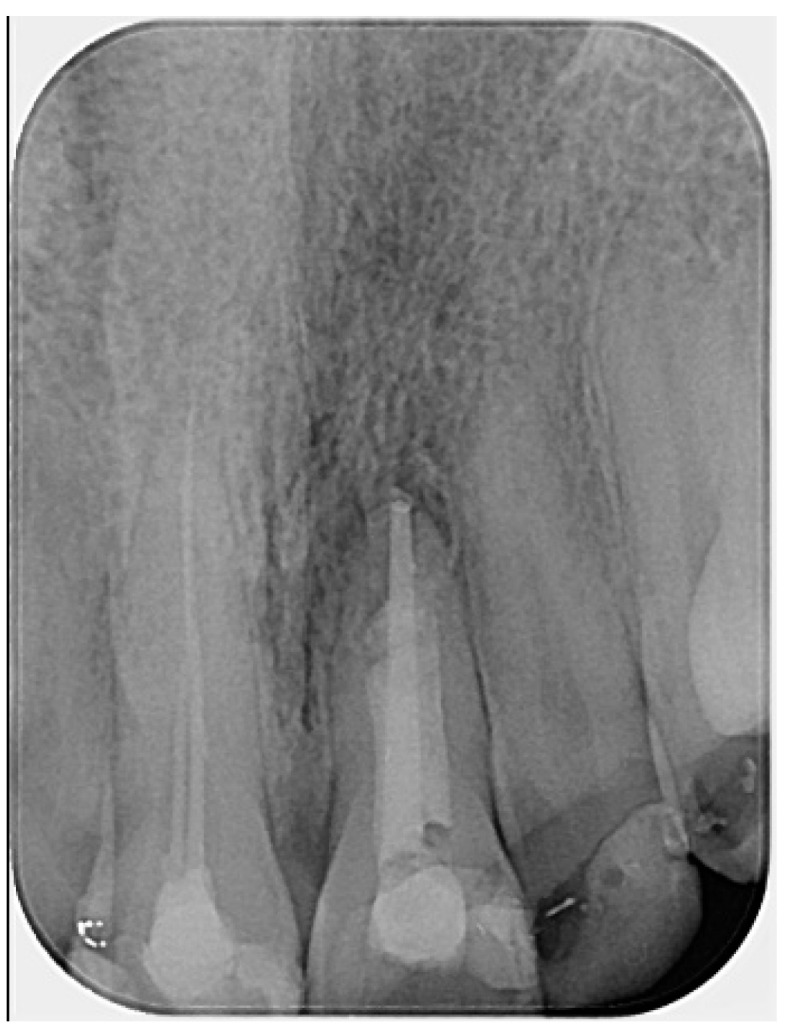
Radiographic verification of the working length confirmed accurate measurement during the clinical appointment. (The mark in the lower left corner indicates the left side.)
